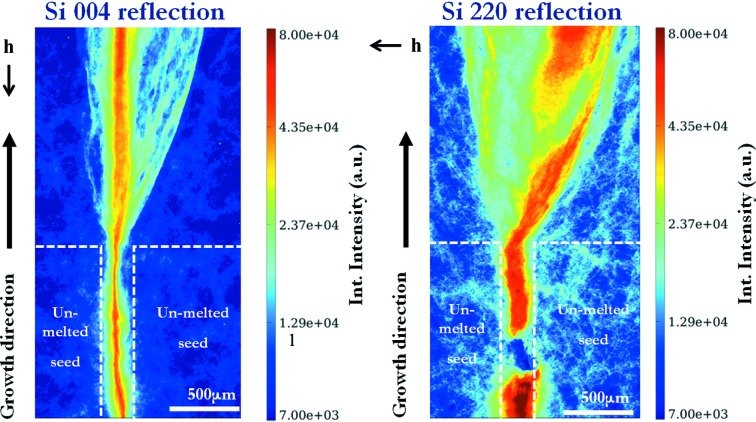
X-ray RCI INT map of the Si 004 and Si 220 reflections from the bottom of the Si ingot at the junction between the two seeds.
